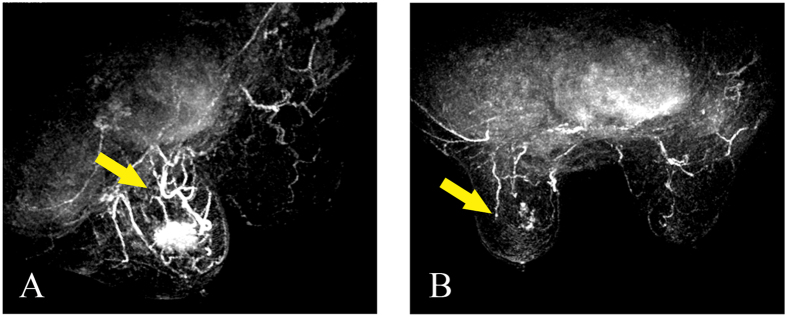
(A,B) Penetrating vessel change on DCE-MRI before and after completion of NAC for breast cancer (female, 57 years old, invasive ductal carcinoma, grade III). (A) Before NAC, DCE-MRI image showed coarse penetrating vessels compared to the contralateral breast. (B) After the completion of NAC, the penetrating vessels and the tumor disappeared.
